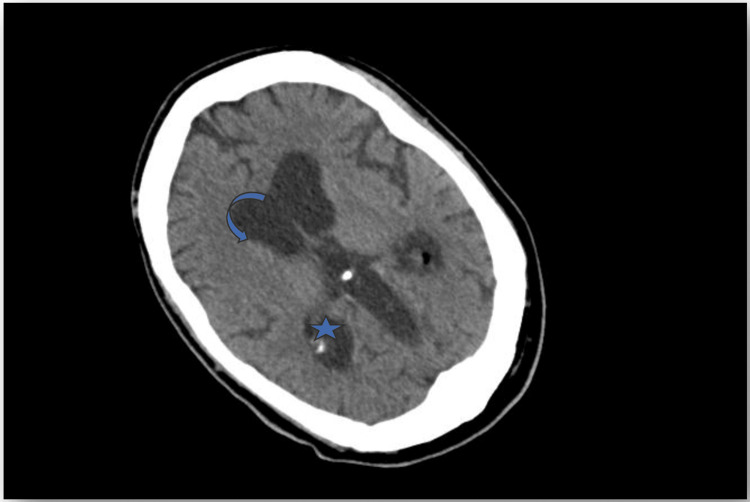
CT brain scan (axial view) of the patient, where features of partial ACC are noted; enlargement of the atria (asterisk), convex-shaped lateral portion of the anterior horns (open circle arrow). ACC: agenesis of the corpus callosum
